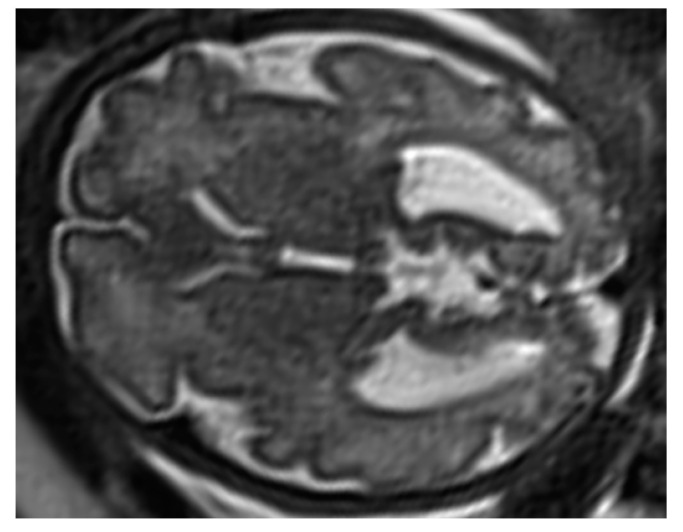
MRI picture of CVS defect.
